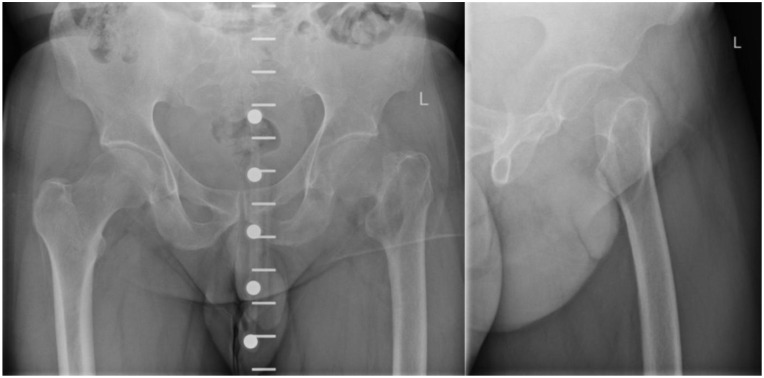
Initial preoperative anteroposterior and additive axial radiograph of the left hip demonstrating an acute, atraumatic pathological fracture of the femoral neck.
